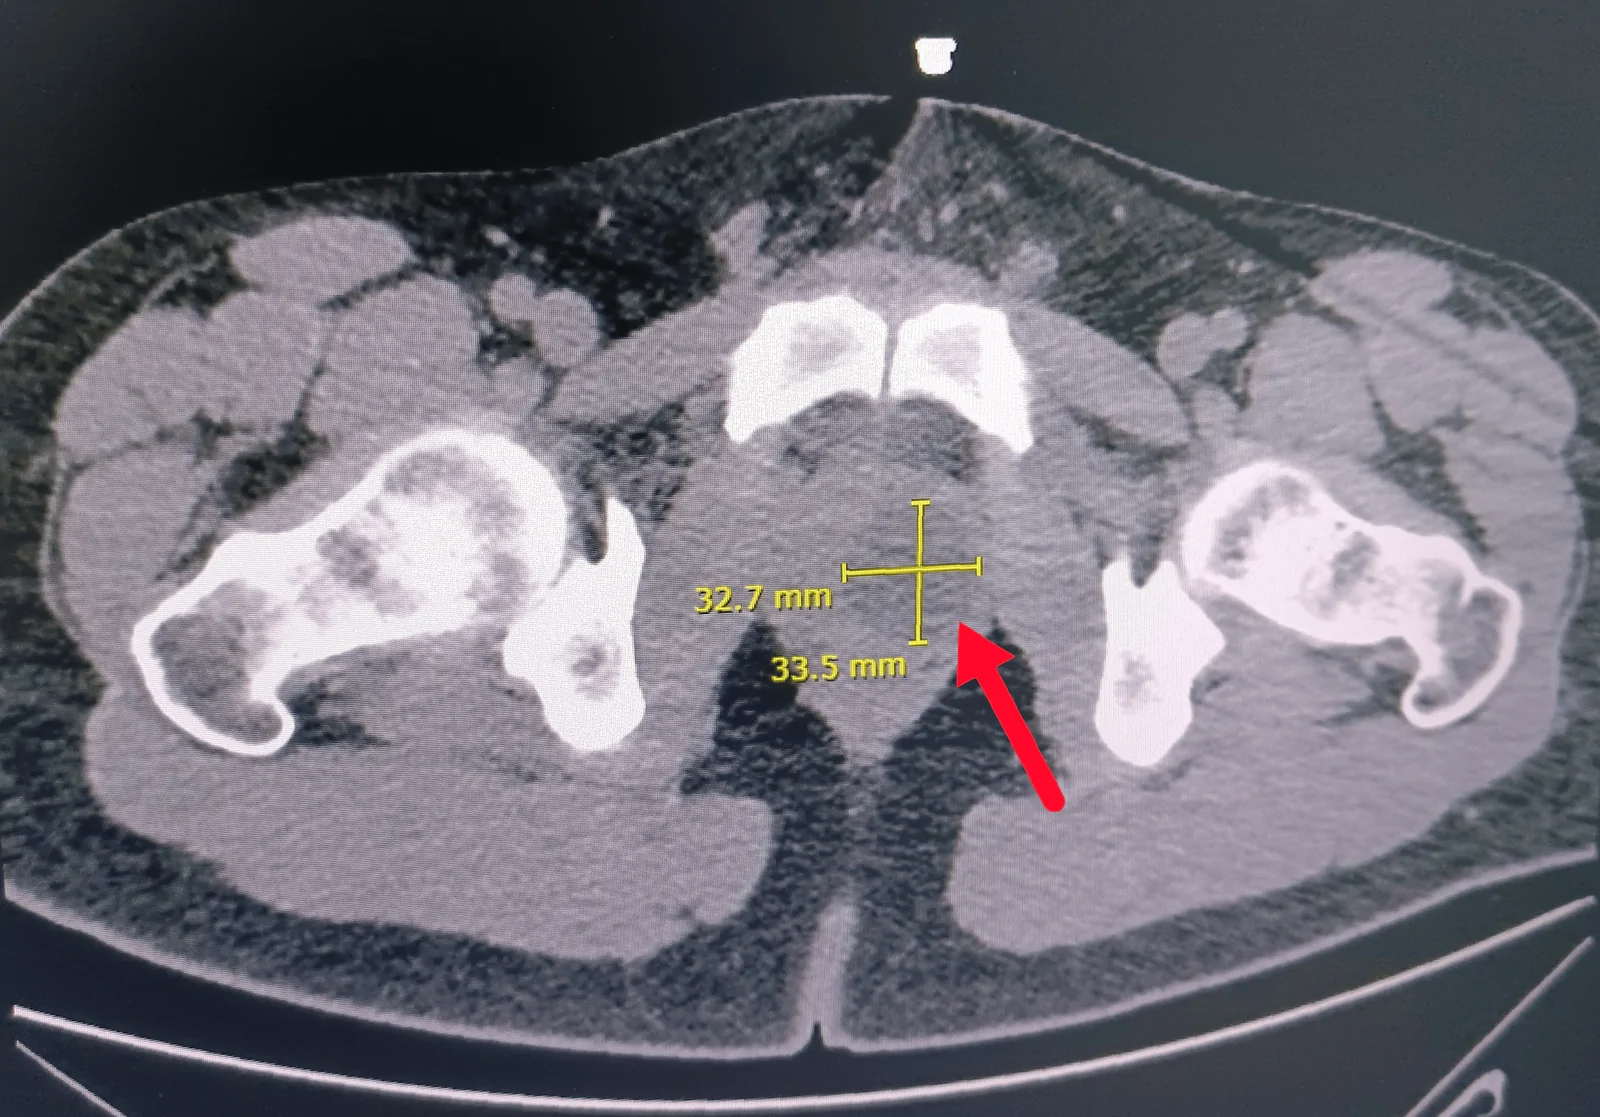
Non-enhanced CT of the pelvis showing the suspicion of a cyst in the left prostatic lobe with its dimensions (red arrow).
